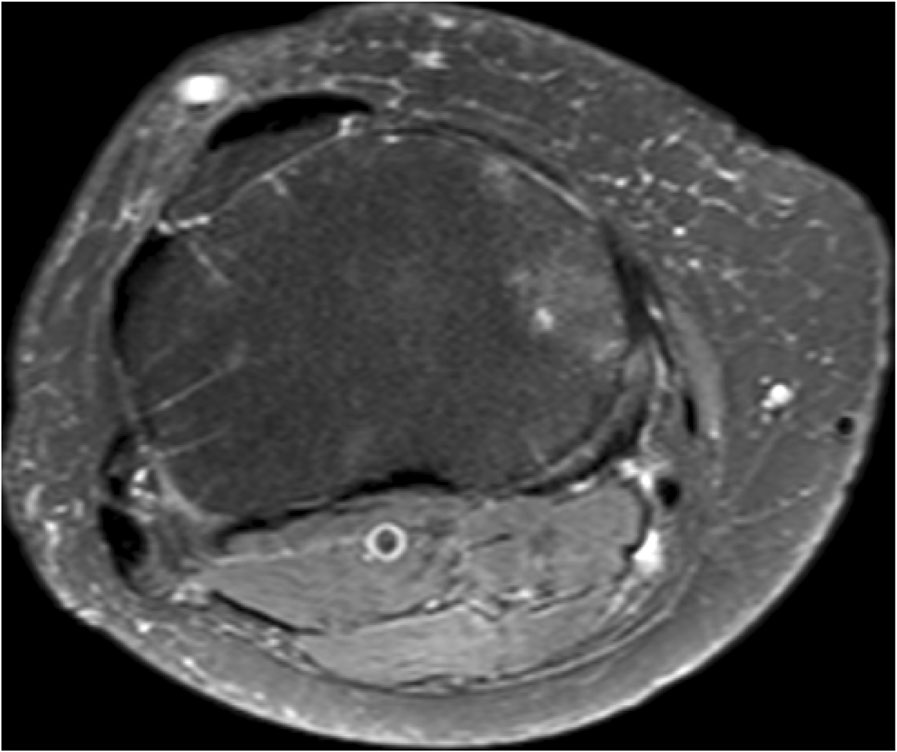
Axial MRI slice with medial OA. A typical slice at level 3, right knee, with K-L grade 2 medial osteoarthritis on plain x-ray. The medial vascular marks are absent while the K-L grade 0 lateral side retains the vascular marks
